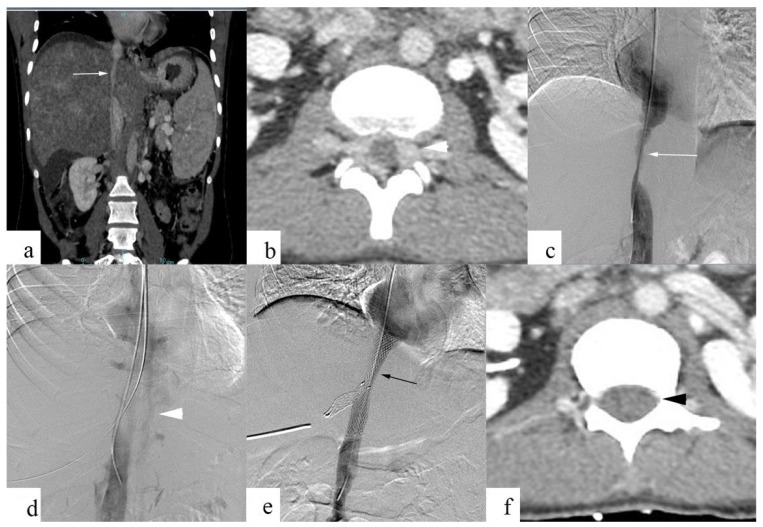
Chronic IVC stenosis in a 32-year-old male patient with Budd–Chiari syndrome and back pain. Contrast-enhanced CT and digital subtraction angiographic images show chronic IVC stenosis involving intrahepatic IVC (a,c; white arrow), and paravertebral venous plexus and epidural venous plexus enlargement (b,d; white arrowhead). Balloon angioplasty, primary stenting, and hepatic venous obstruction (e; black arrow) were performed for IVC, following which the resolution of EVEs (f; black arrowhead) was noted, along with back pain relief.
